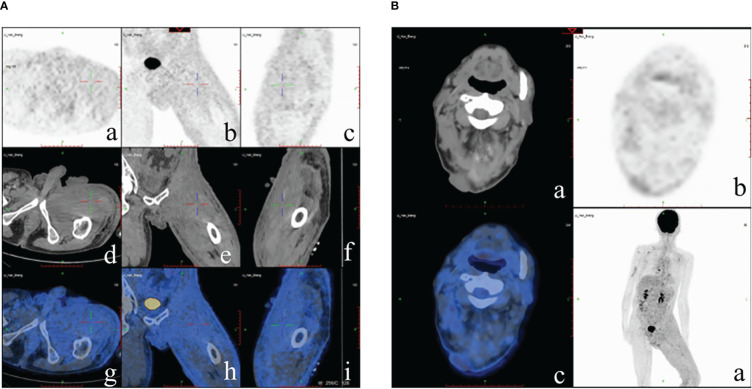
PET-CT examination. (A) Multiple humus-like hypodense nodules on the skin surface from the left groin to the thigh, secondary lymphedema, and slightly increased metabolic diffusion; (B) Localized thickening of the skin on the top of the left head and back of the neck and slightly increased metabolism.
